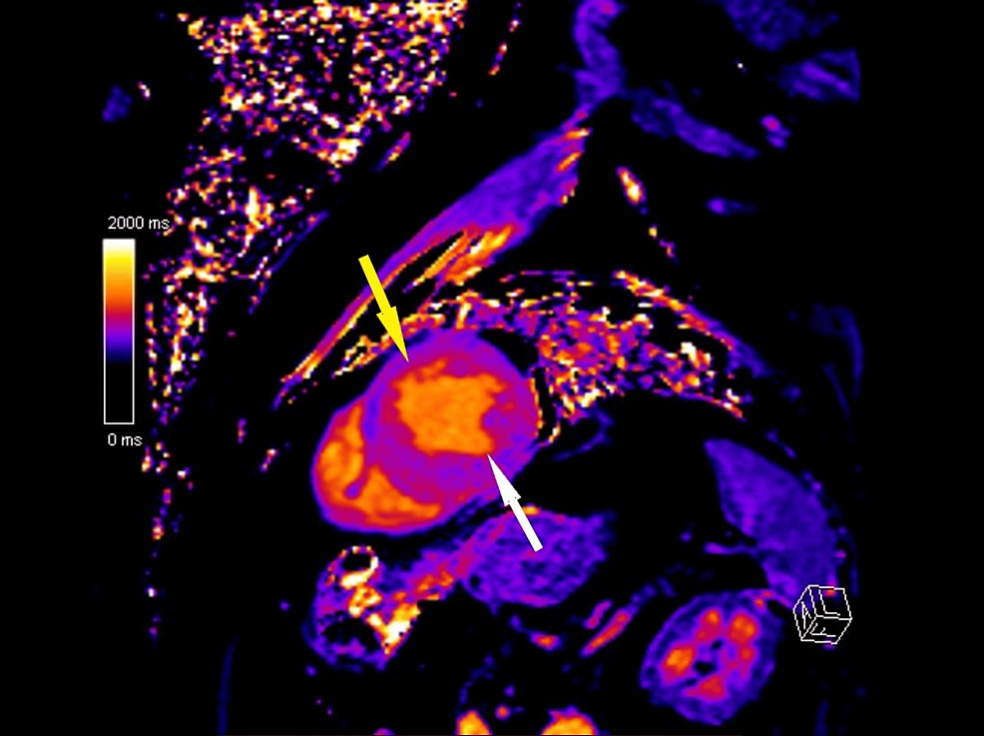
Cardiac magnetic resonance imaging (MRI) T1 map The bright orange area in the myocardium suggests prolonged T1 relaxation time (white arrow). The surrounding purple and blue areas represent normal myocardium with shorter T1 relaxation times (yellow arrow)
